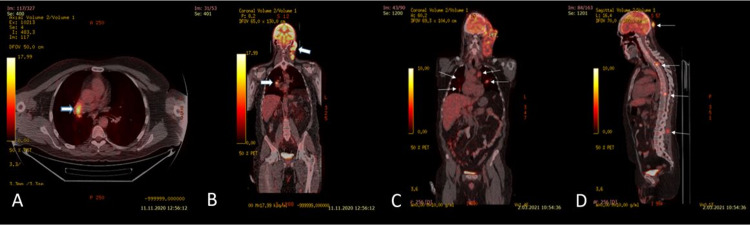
Fused FDG PET/CT scanning conducted seven months after the reoperation revealing an area of hypermetabolism in the right hilar lymph node (arrow) (A) and showing the local progression of the GBM in the left collar region (arrows) (B). (C, D) Repeat fused FDG PET/CT scanning in identifying disseminated metastatic spread to the lungs, mediastinal lymph nodes, scalp, vertebrae, and surrounding paravertebral soft tissues (thin arrows). FDG: Fluorodeoxyglucose; PET-CT: positron emission tomography-computed tomography
